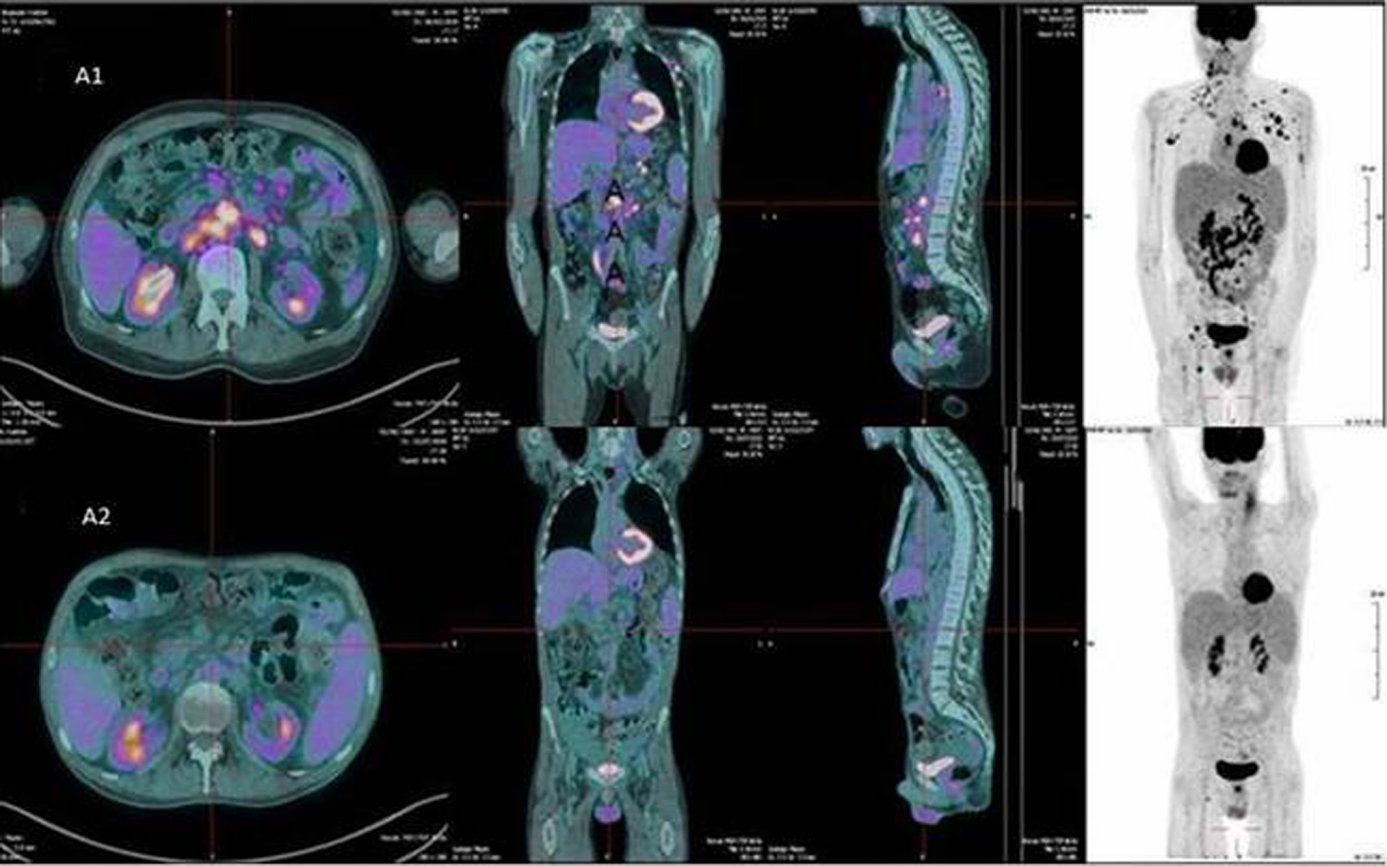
FDG/PET imaging in patient 1. (A) FDG/PET imaging showing complete metabolic response after 3 cycles of AZA+VEN with disappearance of lymph nod and bone lesions. (B) FDG/PET imaging before AZA+VEN initiation showing cervical, axillary, mediastinal, mesenteric, retroperintoneal, and bone involvement. AZA+VEN = azacytidine and venetoclax; FDG/PET = fluorodeoxyglucose positron emission tomography.
